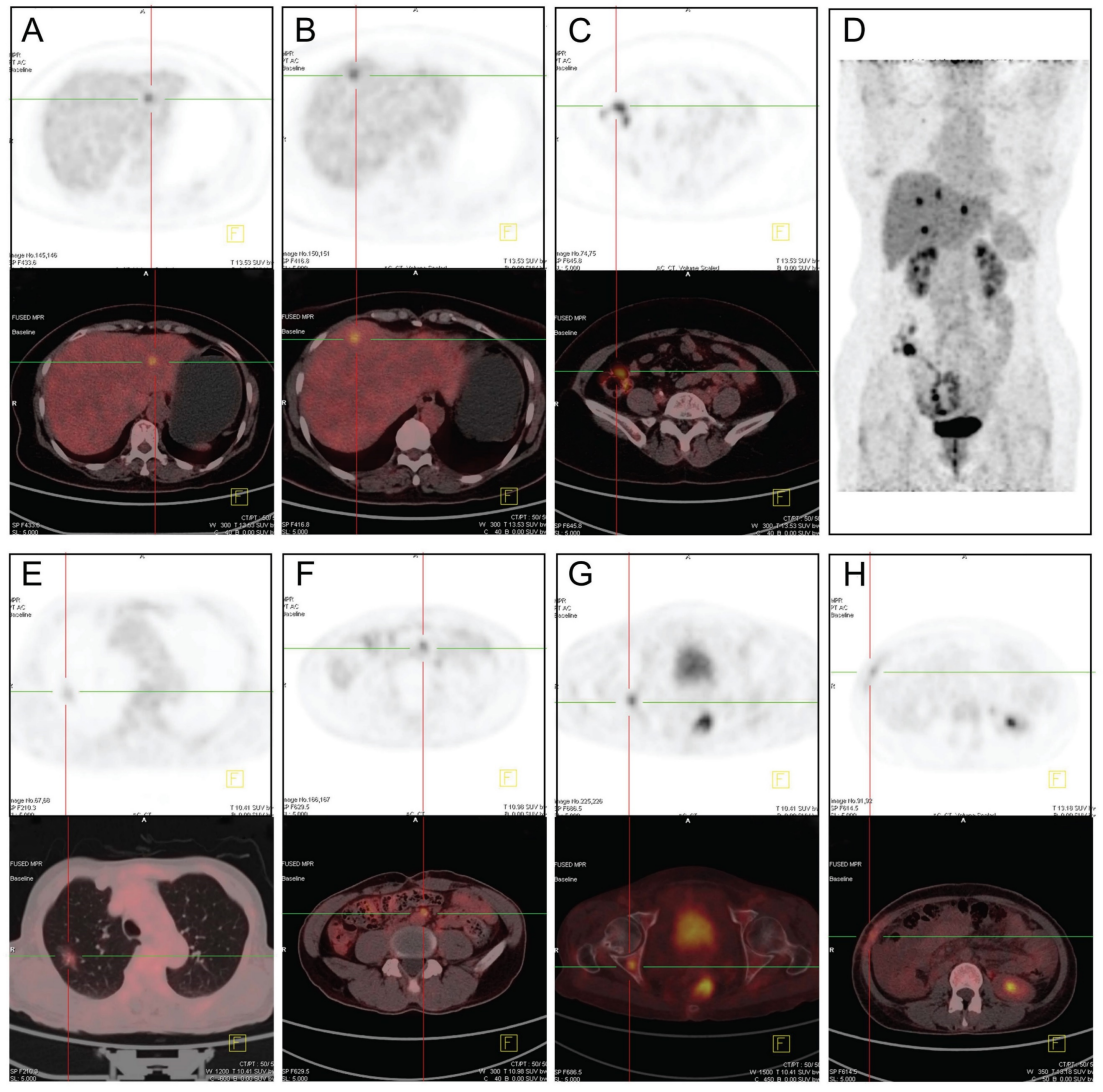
^18^F-FDG PET-CT images of patients with CRLMs and EHMs. (A-D) Different metastatic sites from the same patient. A, SUV_max_ =7.9. B, SUV_max_ =8.5. C, colon cancer with SUV_max_ =9.6. (E) Lung metastases, SUV_max_ = 5.6. (F) Retroperitoneal lymph nodes metastases, SUV_max_= 6.7. (G) Bone metastases on the right acetabulum, SUV_max_= 6.5. (H) Peritoneal and abdominal wall metastases, SUV_max_= 4.9. SUVmax: maximum standardized uptake value.
